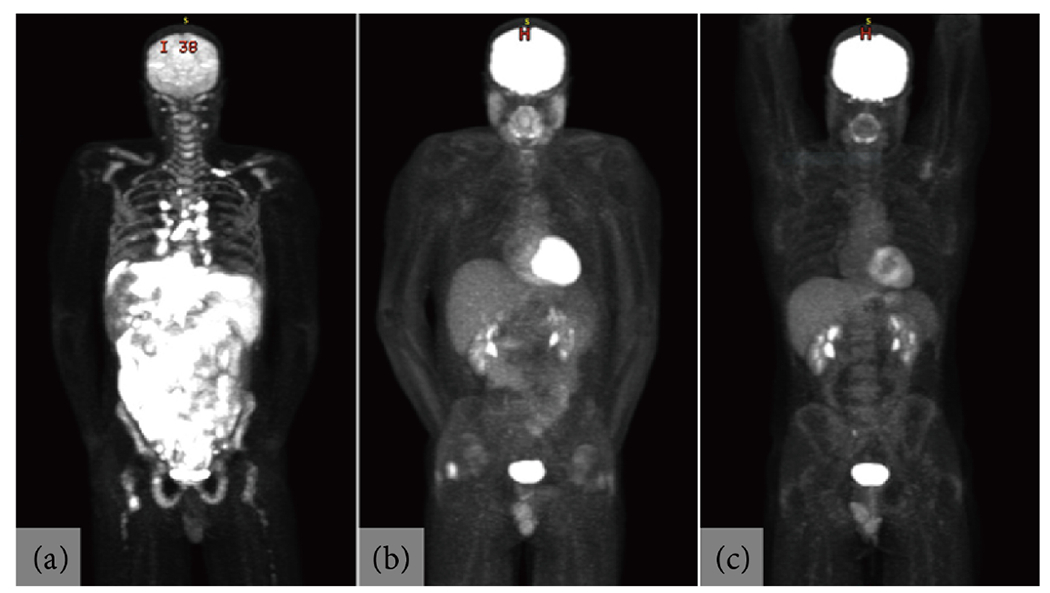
Staging, Interim, and Post-Treatment PET/CT Images. Representative images of PET/CT scans obtained (a) at the time of diagnosis; (b) after two cycles of R-CODOX-M/IVAC, demonstrating a near complete response; and (c) after all four cycles of therapy, demonstrating a complete response (Deauville score 1).
